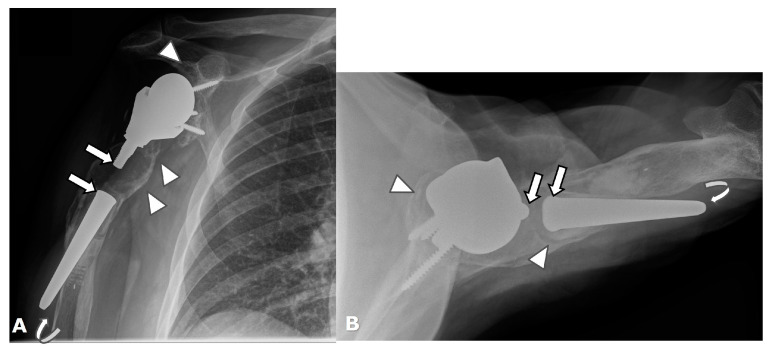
(A) Frontal and (B) axillary radiographs in a patient with RTSA showing failure of the humeral component, now separated into two separate pieces (white arrows). There is significant lucency (arrowheads) along the humeral and glenoid components, with cortical thinning, suggesting loosening or infection. The distal tip of the humeral component (curved arrow) breached the cortex and became extraosseous in location.
